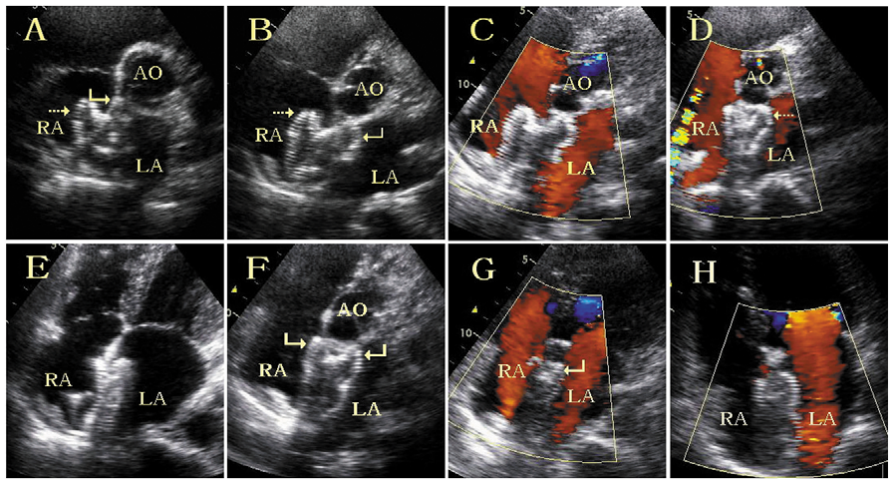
TTE images of the ASO in a patient with ASD with a deficient superior-anterior rim. (A) The left disc upper part was seen to be slipped into the right atrium (curved arrow). (B) After adjusted deployment, the upper parts of the two discs were positioned on the left and right side of the ascending aorta separately and opened as a “Y” shape (dashed and curved arrows). (C) No residual shunt was detected. The cable was pushed to make the right disc upper part press the ascending aorta tightly as shown in the parasternal short-axis view (D) and apical four-chamber view (E). After the ASO was released, the two ASO disc upper parts were positioned on the left and right side of the ascending aorta separately (curved arrows) (F) and no residual shunt was detected as shown in the parasternal short-axis view, the apical five-chamber view (G) and the apical four-chamber view (H). AO = ascending aorta, LA = left atrium, RA = right atrium.
